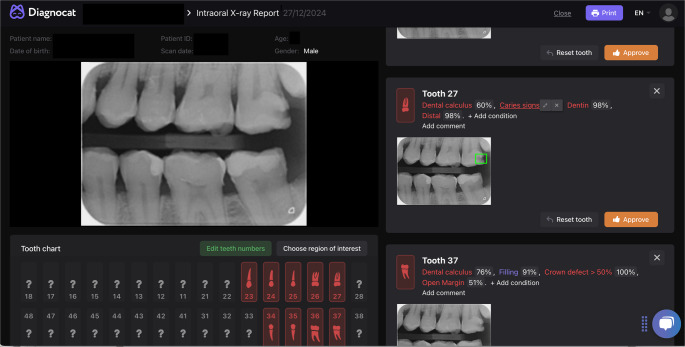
Diagnocat™ output for a bitewing radiograph showing automatic detection of caries lesions. This visualization reflects how the AI software highlights potential caries-related findings. Green-colored overlay highlights the suspected area of decay, with associated probability scores displayed. All other detected pathologies by the software were disregarded for the purposes of this study
